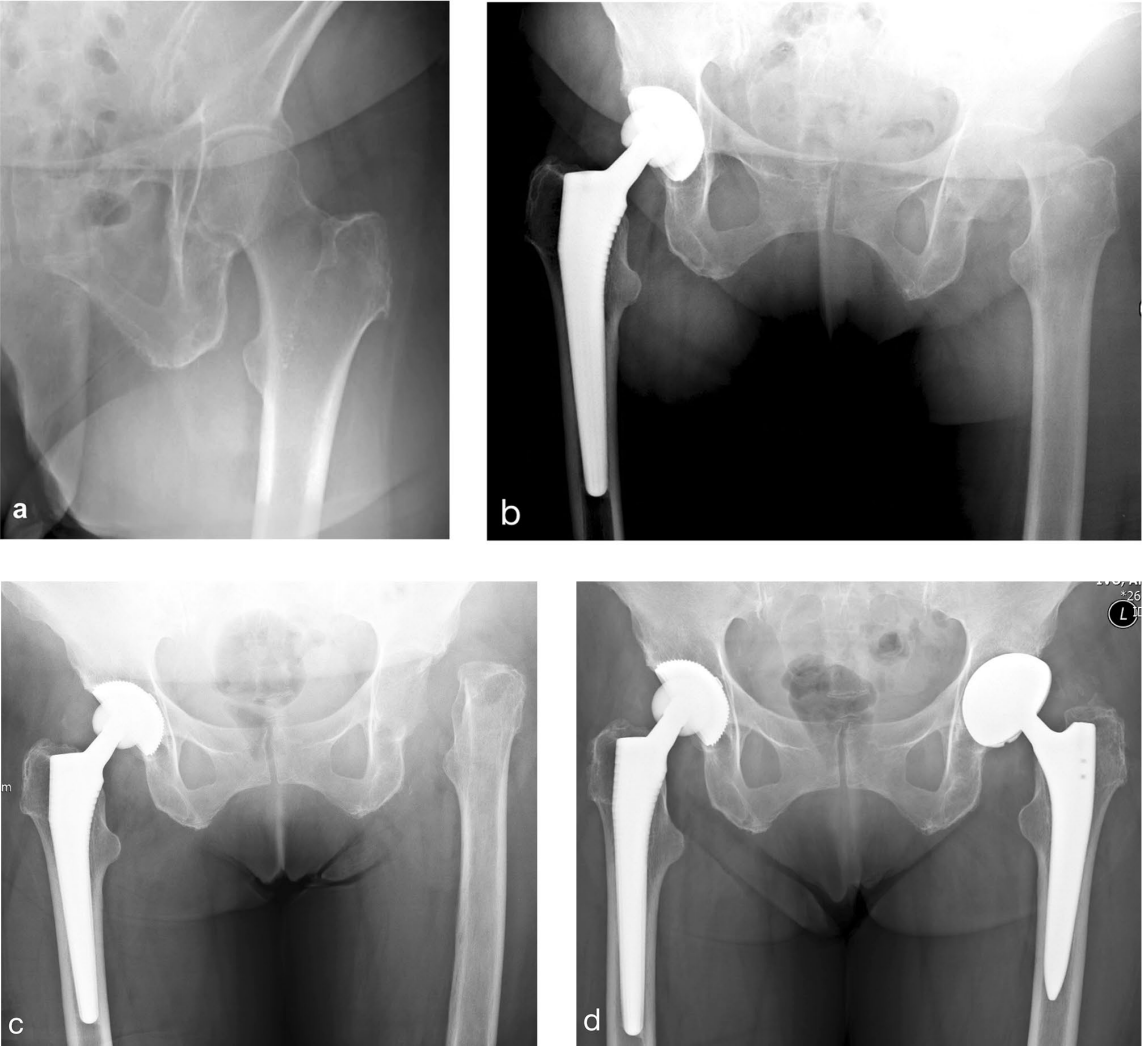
Radiographs of a 79-old female patient with destructive septic arthritis of the left hip. a Anteroposterior view 5 months before clinical presentation. b Preoperative radiograph prior to two-stage total hip arthroplasty (THA). c Postoperative radiograph following resection arthroplasty without spacer insertion. d Postoperative radiograph showing the definitive THA after an interim period of 4 weeks using a highly porous acetabular shell and an extensively porous-coated femoral stem. Leg-length was successfully restored. At latest follow-up, the patient showed no signs of PJI and had a modified Harris hip score of 63 points
